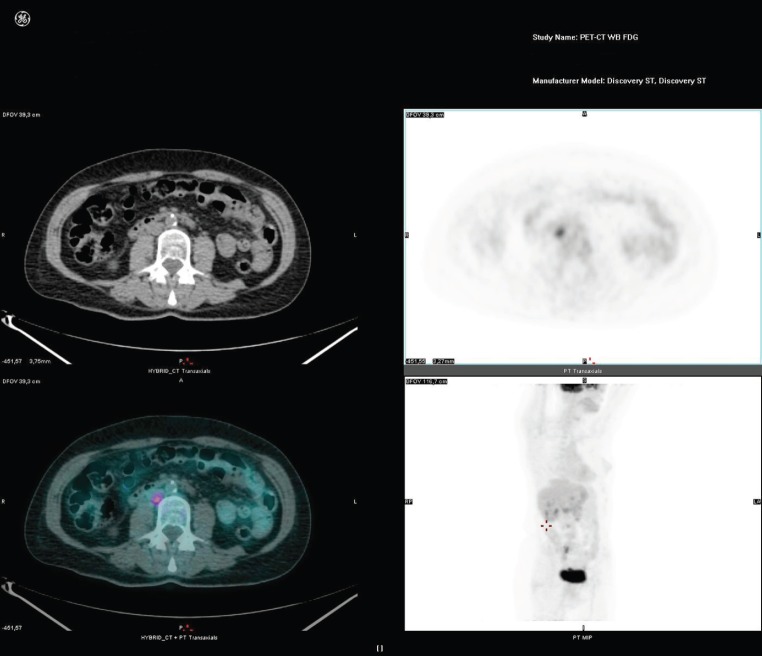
[^18^F]FDG-PET/CT has a higher sensitivity than CT in depicting lymph node metastatic.
